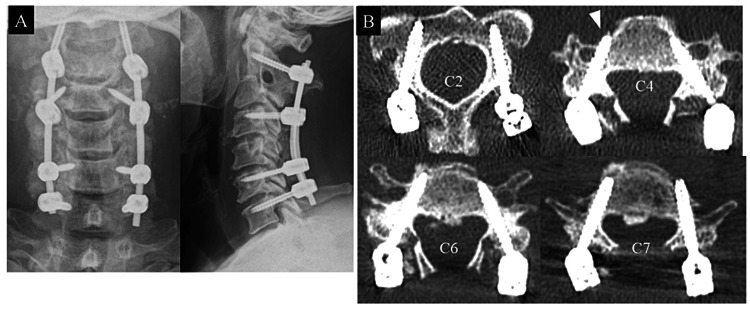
Postoperative imaging findings. A. Postoperative X-ray image. B. Postoperative CT axial image at each cervical pedicle screw (CPS) insertion level. The right side C4 CPS was Grade 1 in the Neo classification, and all the other screws were Grade 0.
